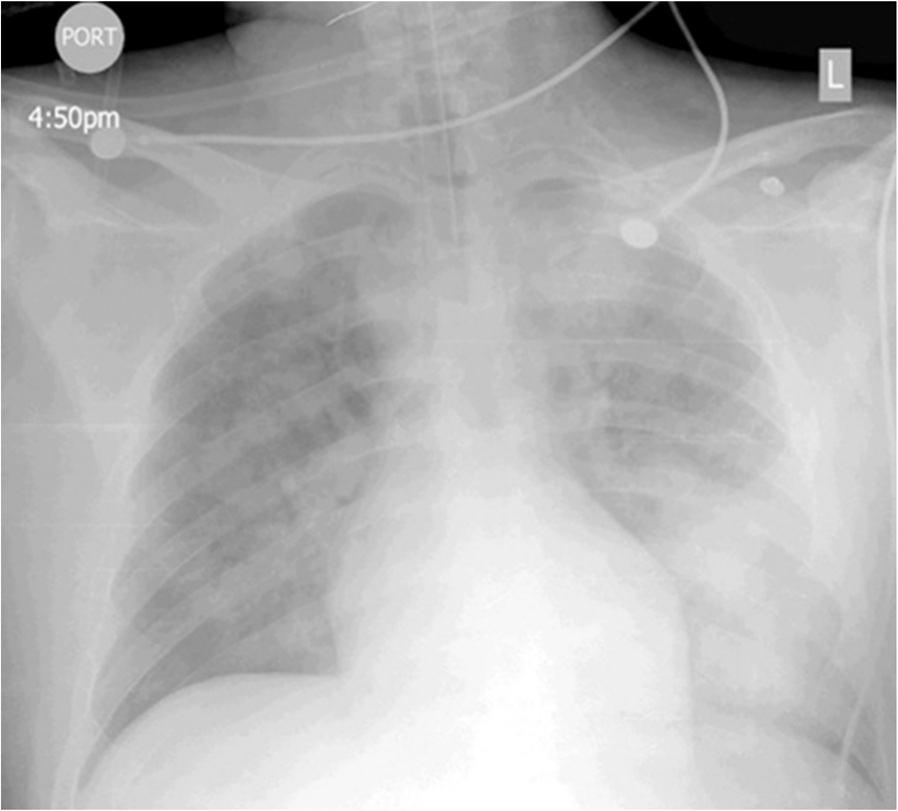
Post-intubation chest X-ray demonstrating diffuse bilateral alveolar infiltrates left more than right.
